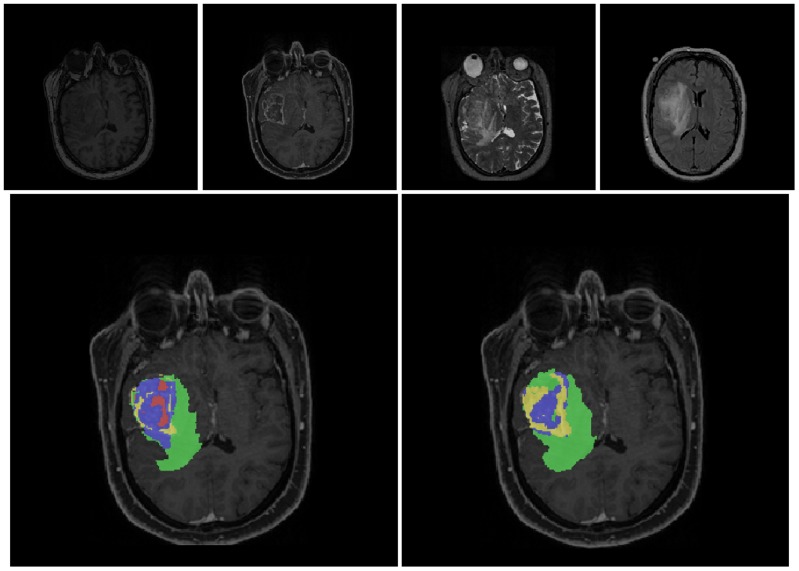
The figures show the original images and the segmentations as overlays on the post-contrast T1-weighted images for a patient with a moderate overlap of manual and automatic segmentation (CETV does not match well). Upper row: an axial slice of the original images (T1w, T1wGd, T2w, FLAIR from left to right). Bottom row left column: manual segmentation, right column: automatic segmentation. Color code for segmentations: red  =  necrosis, yellow  =  enhancing tumor, blue  =  non-enhancing tumor, green  =  edema. TV+ corresponds to a combination of all colors, TV corresponds to red+yellow+blue compartments, CETV corresponds to the yellow compartment.
